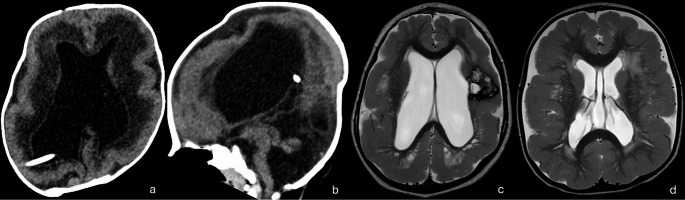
(a, b) Cobblestone Cortex in Walker Warburg Syndrome. Diffuse cobblestone cortex, shunted hydrocephalus with dysmorphic ventricles, and absent septum pellucidum on axial CT (A). Z-shaped configuration of the brainstem and hypoplastic vermis on sagittal reconstruction (B) (c) Cobblestone Cortex in *LAMA2 *mutation*. *Unrelated left periventricular hemorrhagic infarct present (d) Cobblestone-like polymicrogyria in* GPR56 *mutation
